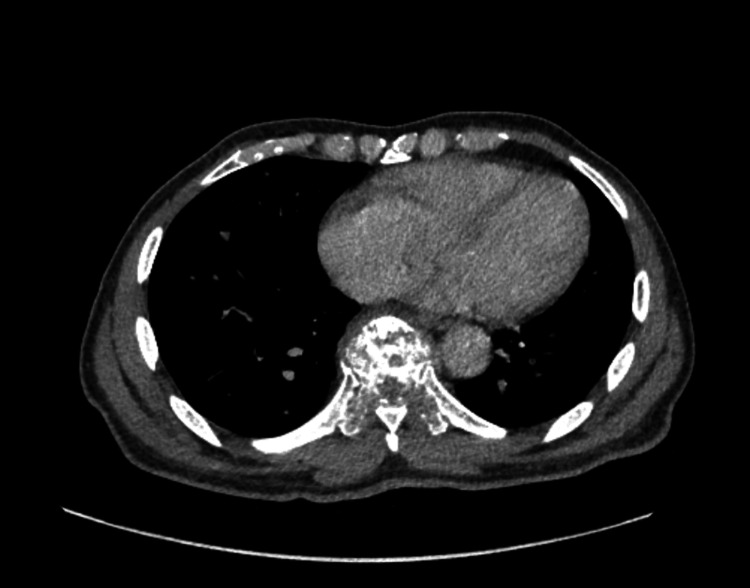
Contrast-enhanced CT scan of the abdomen and pelvis demonstrating lytic destruction of the T9 vertebral body with severe encroachment into the spinal canal
